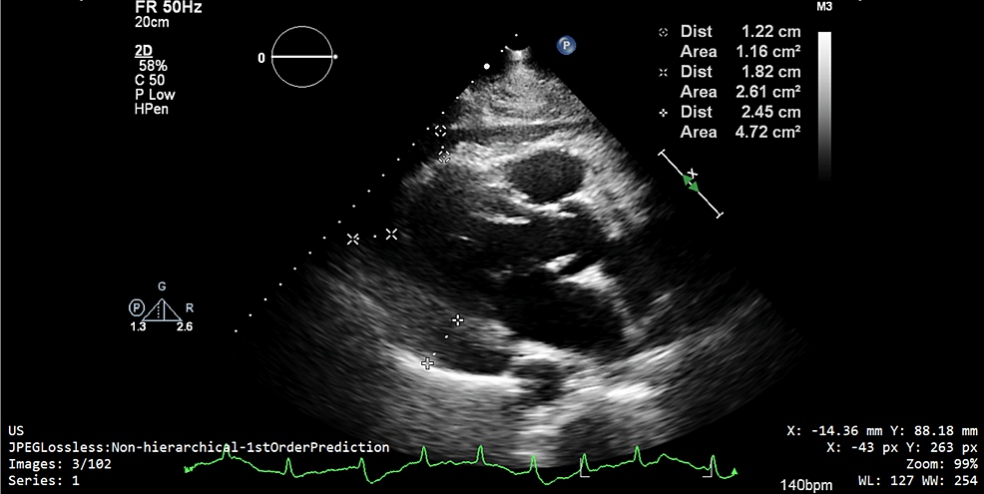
Pericardial effusion seen on echocardiogram, 2.45 cm at its largest
